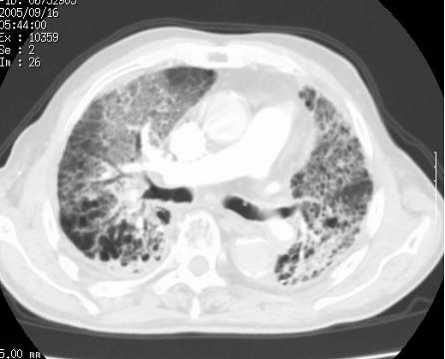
CT images of radiation pneumonitis after SBRT (Pt. No. 5). The finding was acute exacerbation of IP.
